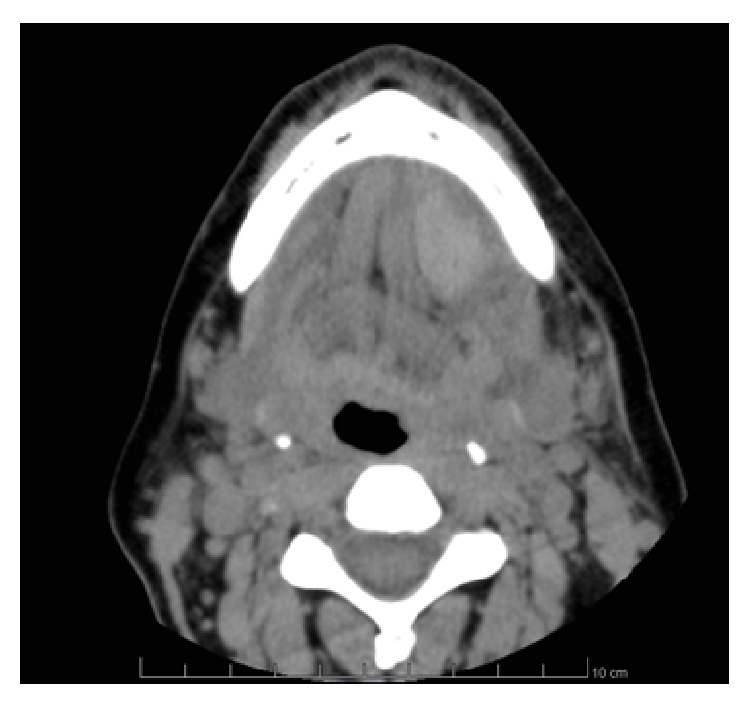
Axial computed tomography image showing almost complete regression of haematoma, normal dimension of upper airways spaces, and normal position of the tongue.
